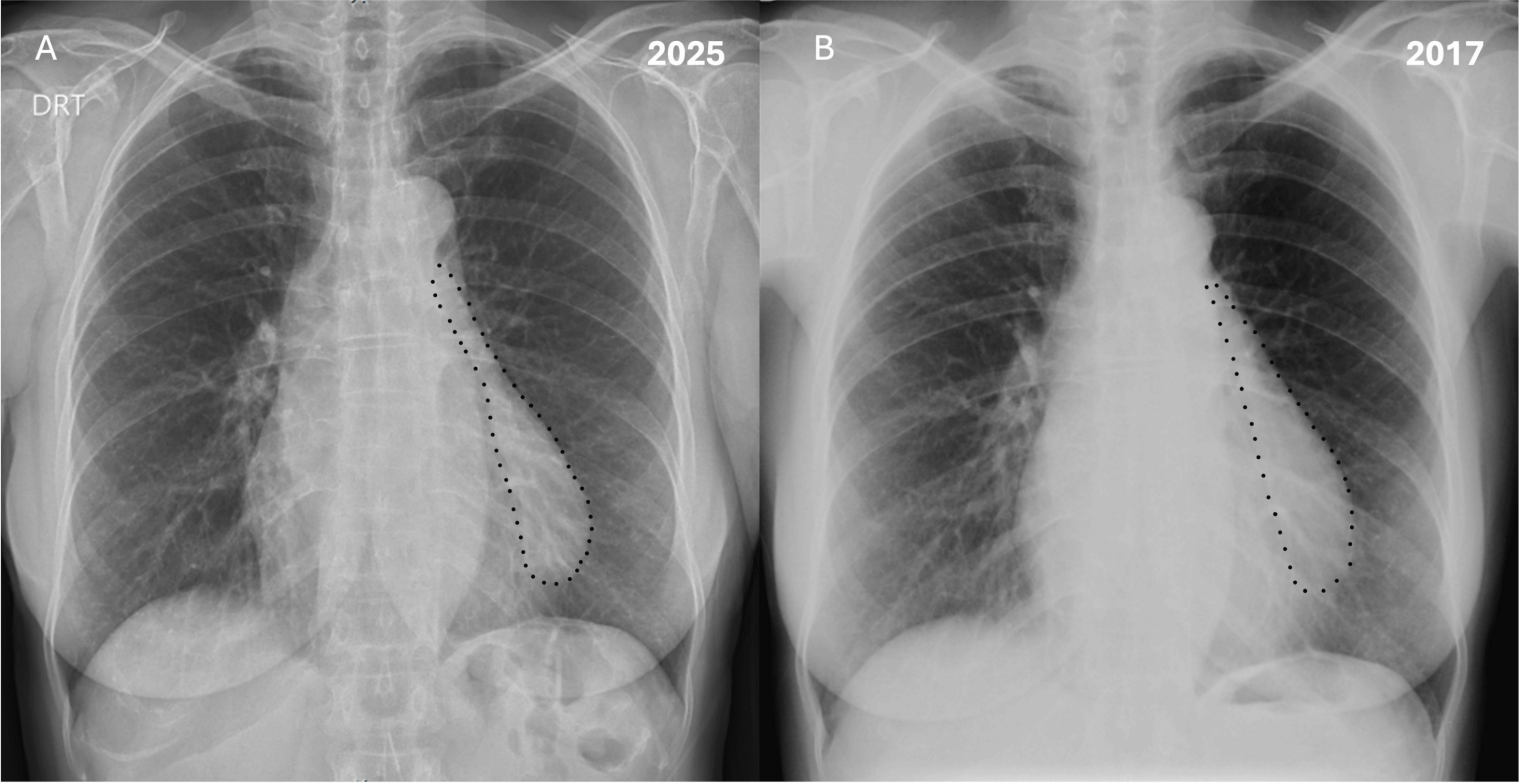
(A) PA chest X-ray showing an enlargement of the upper left heart border, a smooth, well-defined convex opacity, sharply marginated, rounded teardrop-shaped, with clear lung parenchyma. A mass (black dots) is hardly depicted. (B) Retrospectively, in a PA chest X-ray taken eight years earlier, it is evident that the opacity in the left cardiac border, marked by a dotted line, was already present, which reveals no significant interval change in the appearance, size, or location, morphologically and chronologically consistent with a possible benign, stable pericardial cyst. PA: posteroanterior
